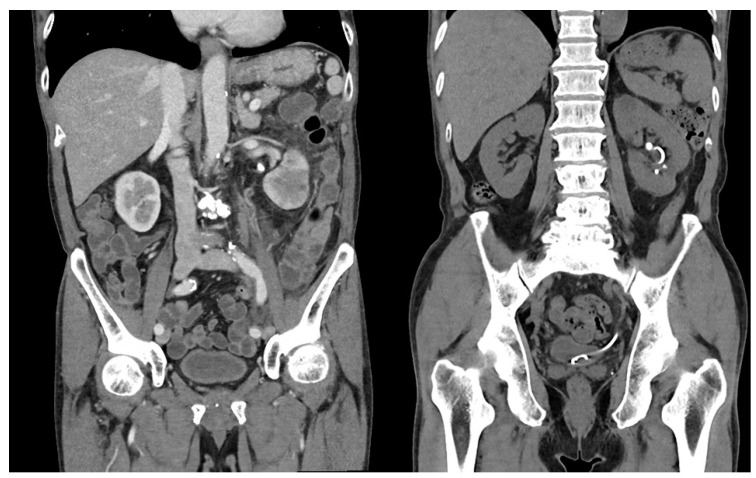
Initial and preoperative CT images of a patient presenting with a proximal ureteral stone with infection. In the right image, the stone migrated to the upper calyx with the inserted ureteral stent. This patient had an mS-ReSC score of 5 (one stone in the renal pelvis and two stones in the inferior minor calyces).
